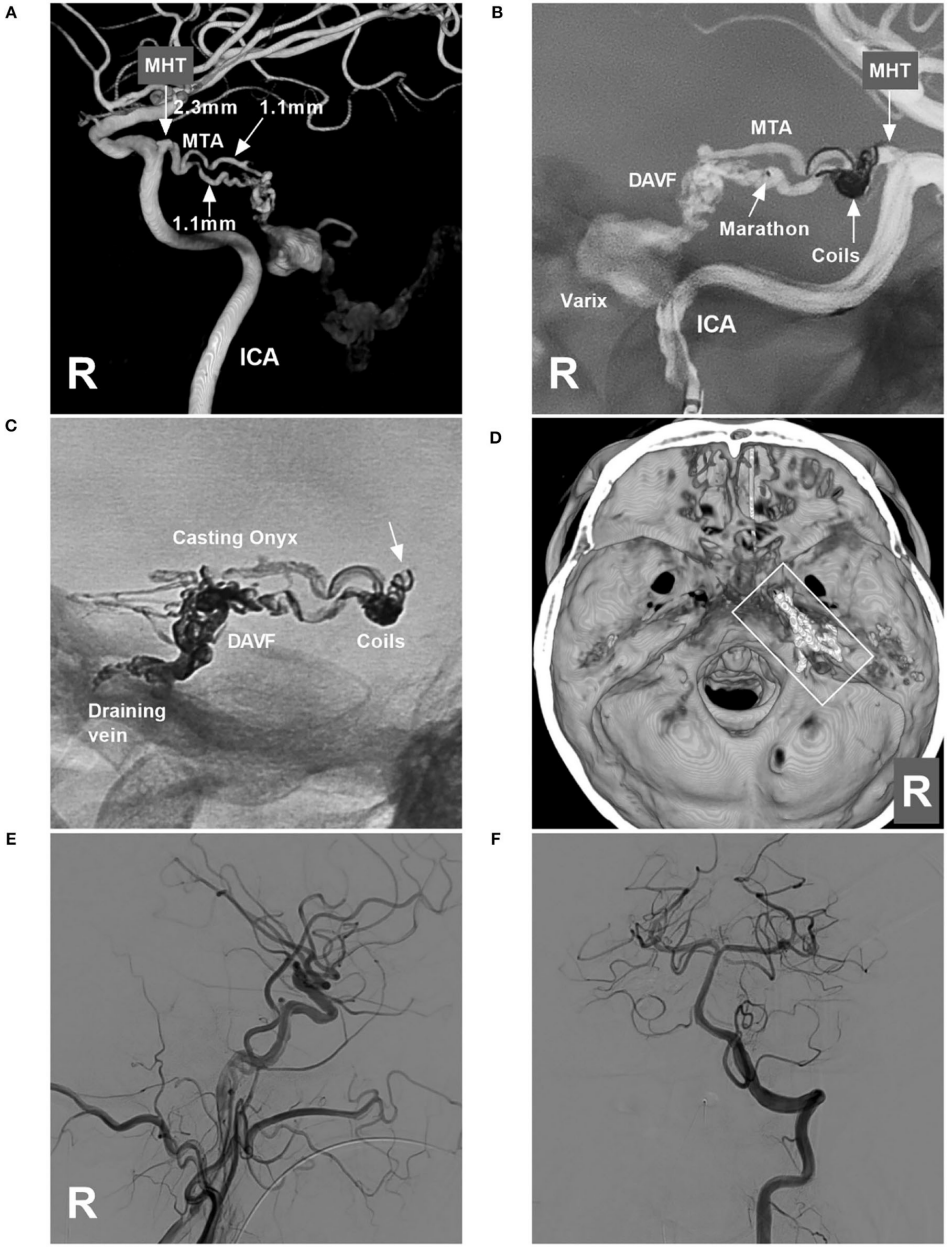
Operative images in case 1. (A) Three-dimensional reconstruction of DSA of the right ICA showing the diameters of the feeding artery to the TDAVF. The MHT origin was 2.3 mm, and the MTA of Bernasconi-Cassinari was 1.1 mm in diameter. (B) Road map of the ICA showing a Marathon microcatheter placed in the lower MTA. The MHT trunk with an aneurysmal dilation was coiled to produce the mass effect. (C) X-ray film showing the coils and casting Onyx. Onyx reflux reached the coils (arrow) but did not extend beyond the coils. (D) Three-dimensional reconstruction of Xper-CT showing the location of the Onyx casting (frame) at the margin of the tentorium. (E,F) DSAs of the right carotid artery (E) and VA (F) show complete obliteration of the DAVF. DAVF, dural arteriovenous fistula; DSA, digital subtraction angiography; ICA, internal carotid artery; MHT, meningohypophyseal trunk; R, right; MTA, medial tentorial artery; VA, vertebral artery.
